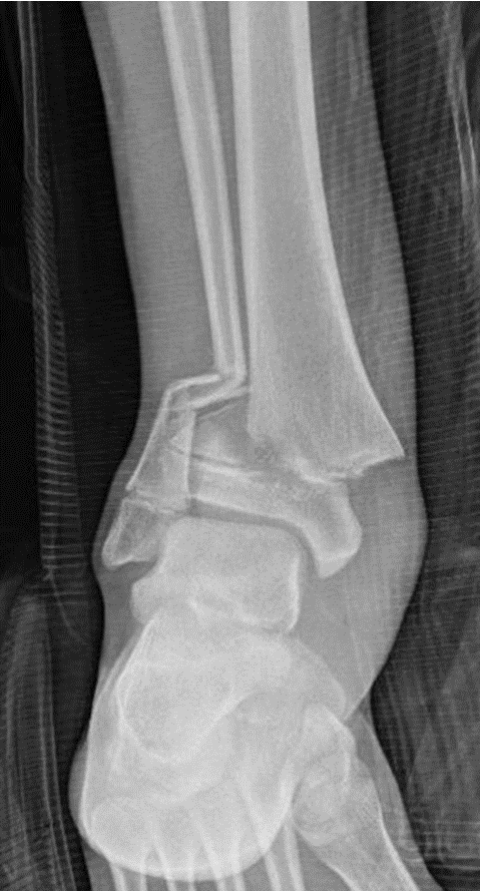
Plain radiograph of a distal tibia and fibula fracture. A plain radiograph of a distal tibia and fibula fracture involving the physis (growth plate) in a 14-year-old individual.
